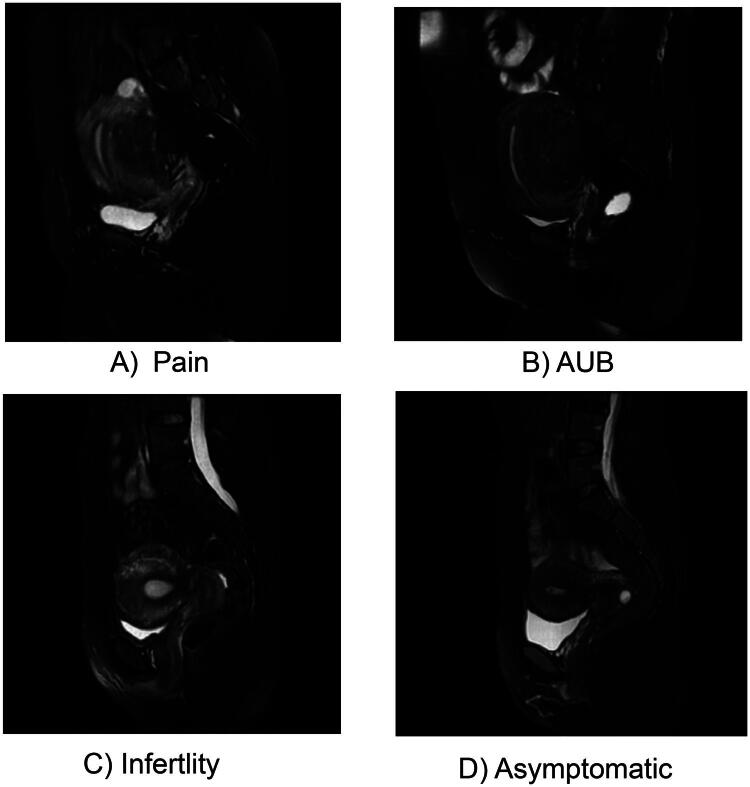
MRI Images of four clinical symptom subtypes of extrinsic adenomyosis. A) Pain, B) AUB, C) infertility, D) asymptomatic. The axial oblique plane was perpendicular to the endometrial cavity, resulting in a short-axis view.
